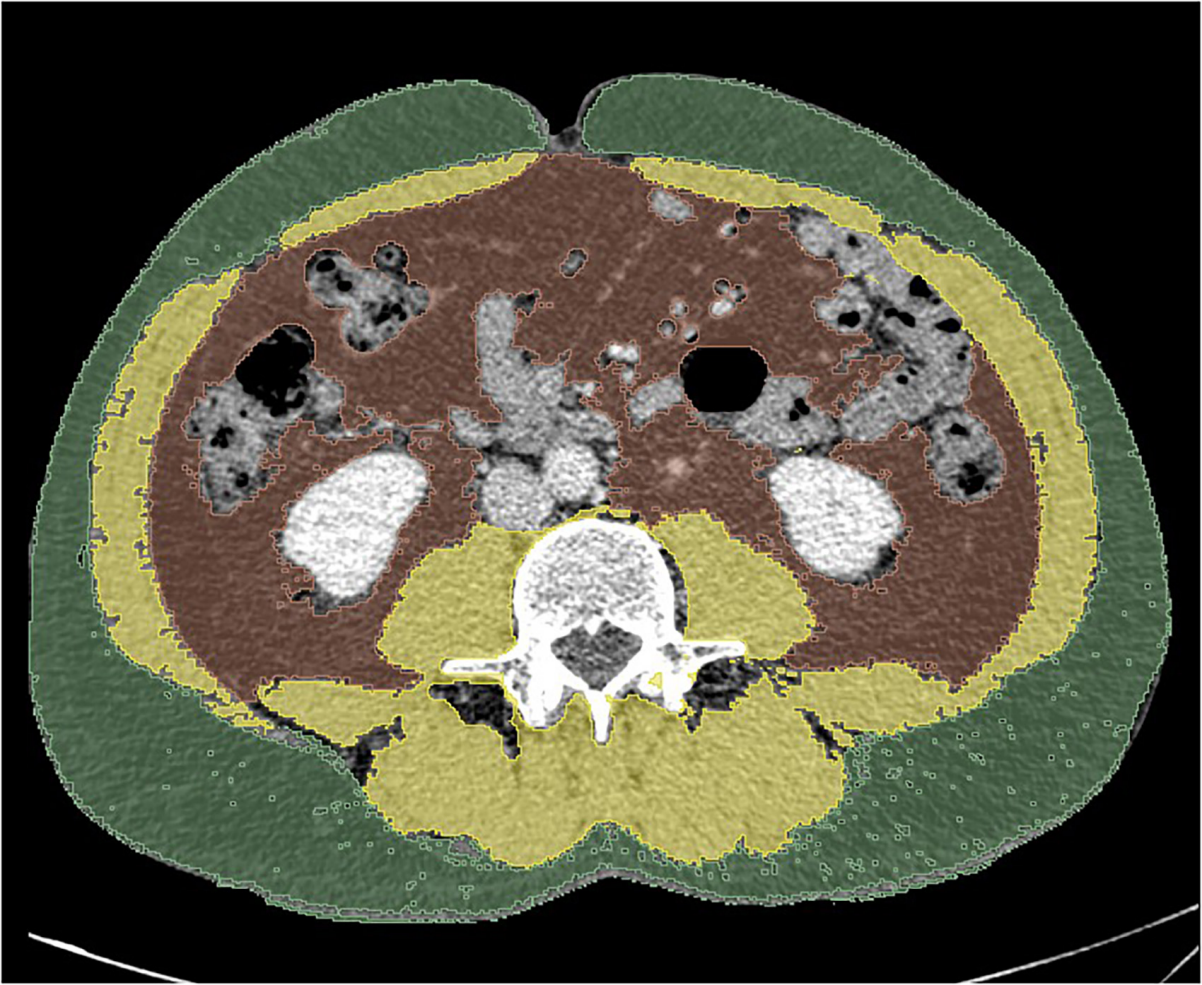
Measurement of body composition parameters on an axial CT slice at the L3 level. Subcutaneous fat area (green), skeletal muscle area (yellow), and visceral fat area (brown).
